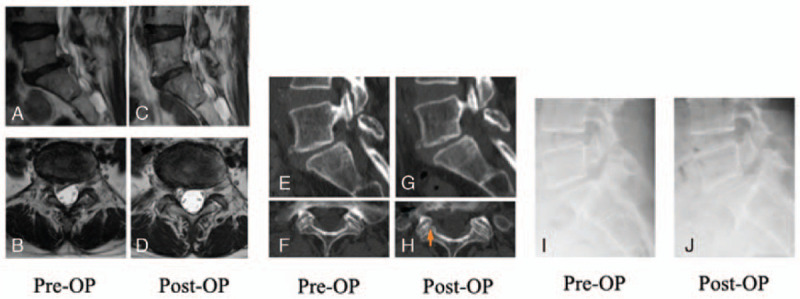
A 51-year-old female who had disc herniation at L5/1 and concomitant L5 spondylolisthesis underwent Full endoscopic discectomy via the interlaminar space (FESS-IL) for L5/1. At 1 year after surgery, there was no sign of recurrence of herniation or progression of spondylolisthesis (I and J). Preoperative sagittal (A) and axial (B) images on T2-weighted magnetic resonance imaging (MRI), and sagittal and axial images on computed tomography (CT) (E&F). Postoperative (1 year) sagittal (C) and axial (D) images on T2-weighted MRI, and sagittal and axial images on CT (G&H).
